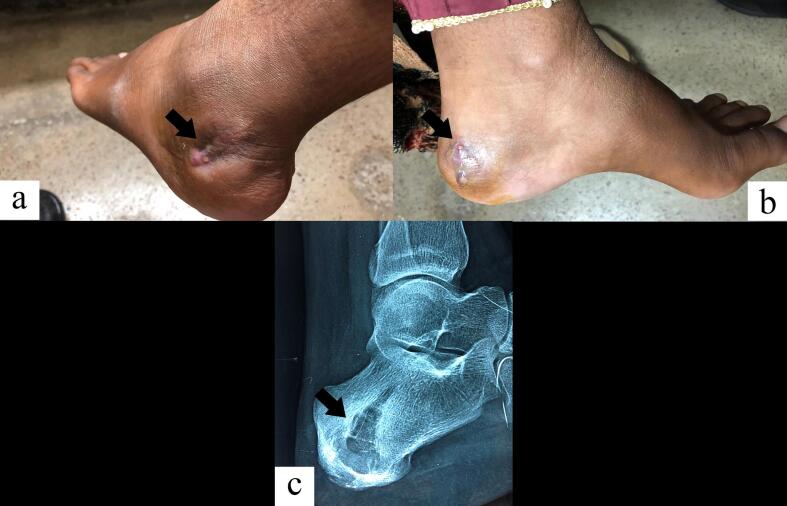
Draining sinuses seen on either side of the posteroinferolateral aspect of the left ankle (A, B). An enlarged osteolytic lesion with a cortical break seen in lateral radiograph of the left foot in the same region (C).
